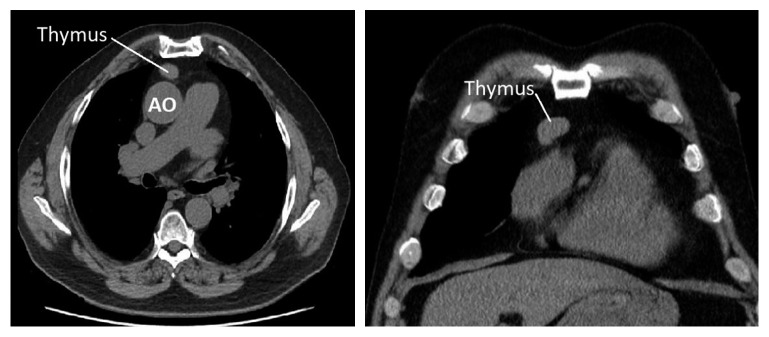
Thymoma on noncontrast chest computed tomography.
